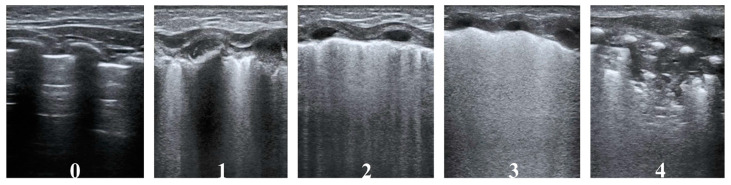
Description of the LUS. The 12-zone scanning protocol was adopted. For each lung area, a score from 0 to 4 was assigned. Score values corresponded to 5 different patterns, as shown in the ultrasonograms. The score was given as follows: 0 points (presence of <3 well-spaced B-lines); 1 point (presence of ≥3 well-spaced B-lines); 2 points (B-lines are difficult to count, or partially coalescent); 3 points (fully coalescent B-Lines, with or without minor consolidations limited to the subpleural space); 4 points (extended consolidations).
